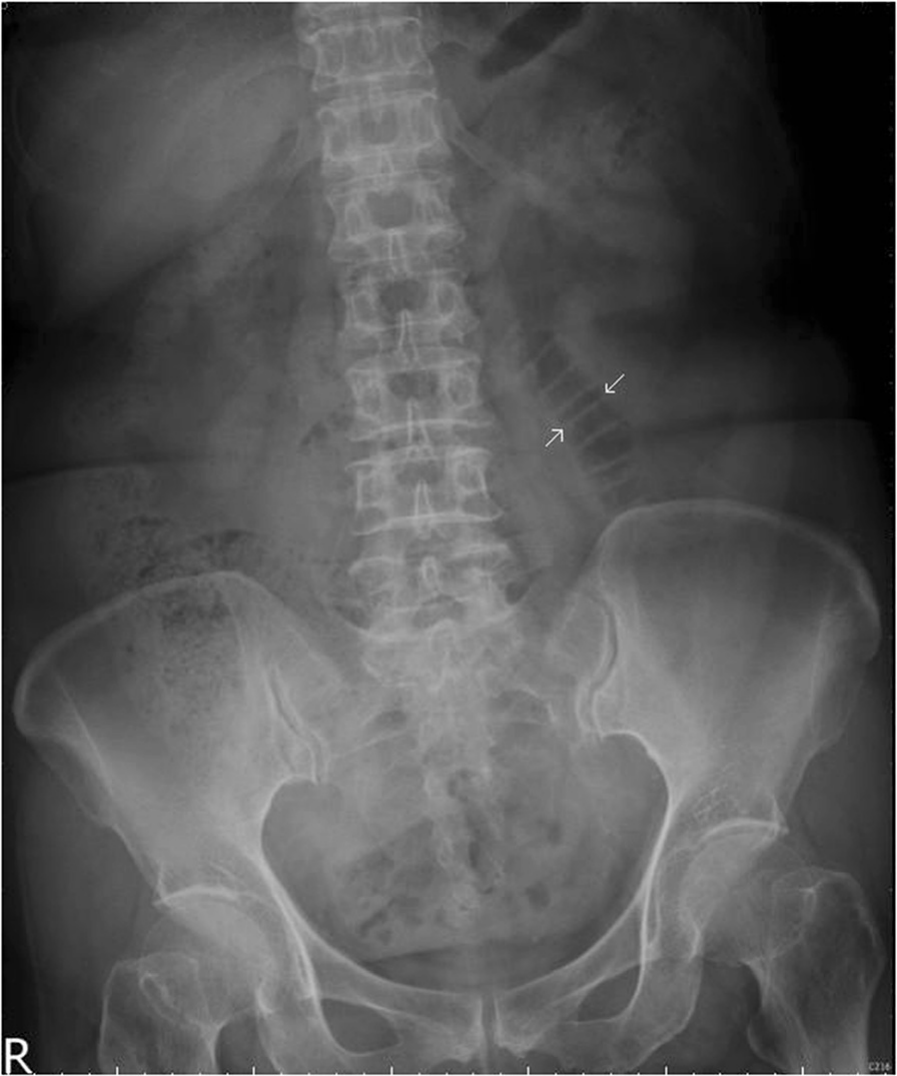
Plain film findings. A solitary dilated loop of small bowel was in the left upper quadrant (arrow).
